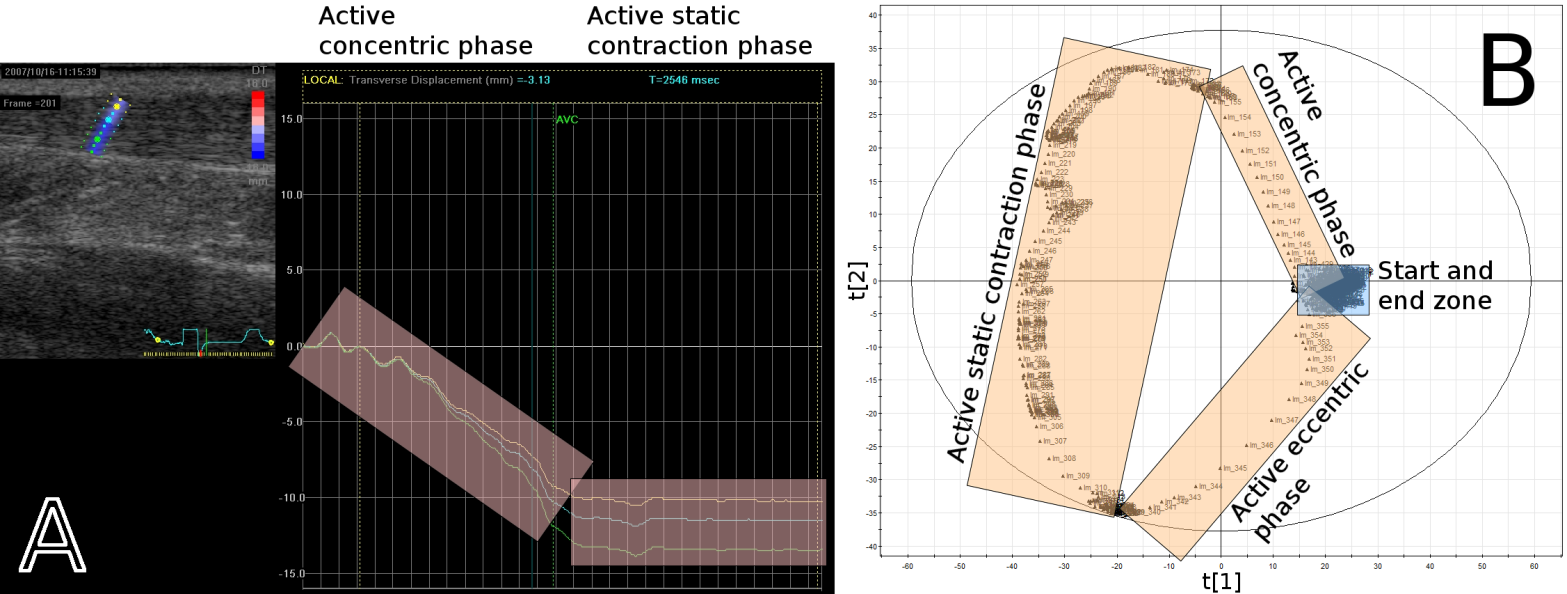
The results of case example 2. (a) The plantar flexion movement starts at rest and enters the active concentric phase, which is transformed into a stationary phase, the active static contraction phase, and ends with the active eccentric phase, returning to rest. The last phase is missing in the figure because of a time limitation in the commercial ultrasound software. The eccentric phase is, however, confirmed if the contraction phase is cropped and only the stationary and eccentric phases are examined, or equivalently if the in-house developed speckle tracking software is used. The PCA score plot (b), where the second score vector, t2, is plotted against the first score vector, t1, shows that the start and end zones of the movement are located in the same area of the plot, implying a relaxed muscle before and after the MVC.
